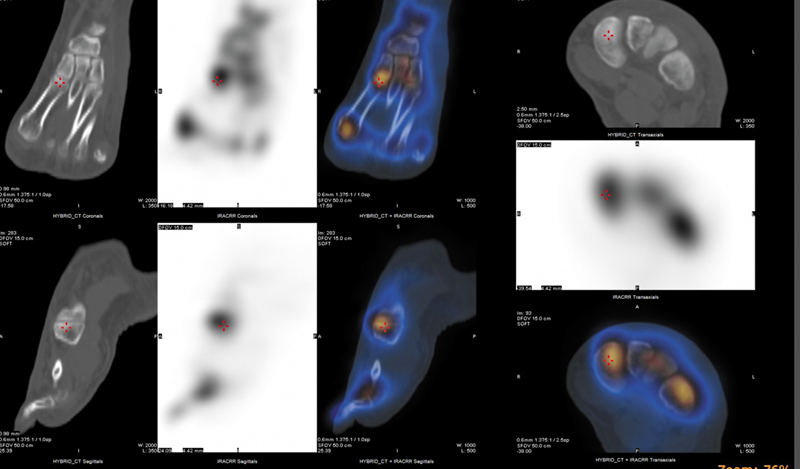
SPECT/CT imaging of the left foot. The figure includes CT images, nuclear medicine images, and fused hybrid SPECT/CT images, demonstrating localized tracer uptake and corresponding anatomical abnormalities in the affected region. CT, computed tomography; SPECT, single-photon emission computed tomography.
